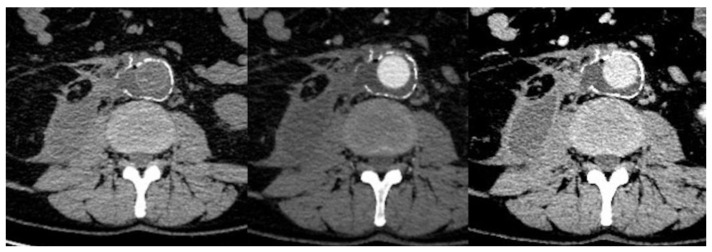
Open surgical repair of abdominal aortic aneurysm (65-year-old male). From left panel to right: unenhanced and enhanced (arterial phase and late phase) CT scans 4 months after treatment show aortic graft patency with perigraft fluid and air, enhancing the soft tissue around the graft and abscess near the right psoas muscle. These findings are consistent with perigraft infection, as also confirmed by fluid aspiration.
